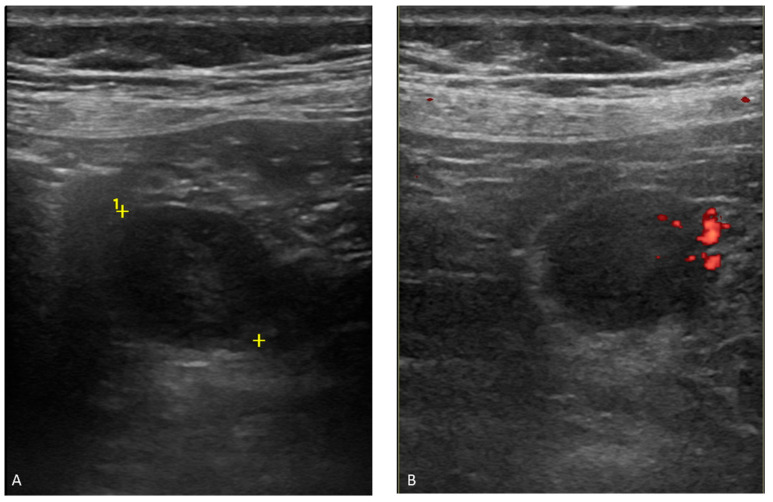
Abdominal ultrasound (US): (A) US mode; and (B) color Doppler. Hypoechoic area (2.3 cm), well delimited, at the jejunal level. Hypoechogenic tumoral mass, well delimited and with vascular pedicle.
